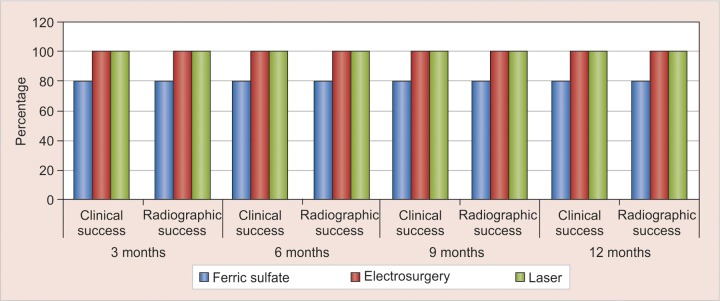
Overall clinical and radiographic follow-up at 3, 6, 9 and 12 months
